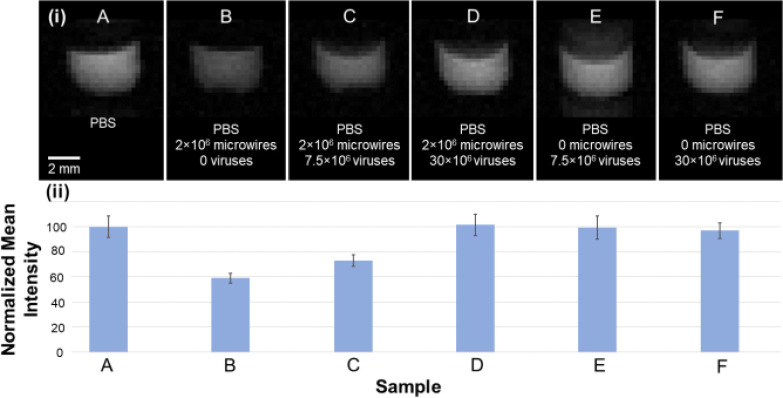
(i) MR images of 50 μl PBS samples with various concentrations of micro-devices. (A) No microdevices, no viruses. (B) Low intensity seen after adding 2 × 10^6^ micro-devices, no viruses. (C) Reduced intensity with 2 × 10^6^ micro-devices, 7.5 × 10^7^ copies/ml. (D) Recovery of intensity with 2 × 10^6^ micro-devices, 30 × 10^7^ copies/ml. Control images on samples having only viruses (E, F) suggest that viral content alone, at the concentrations shown, is insufficient to appreciably alter mean intensity.
